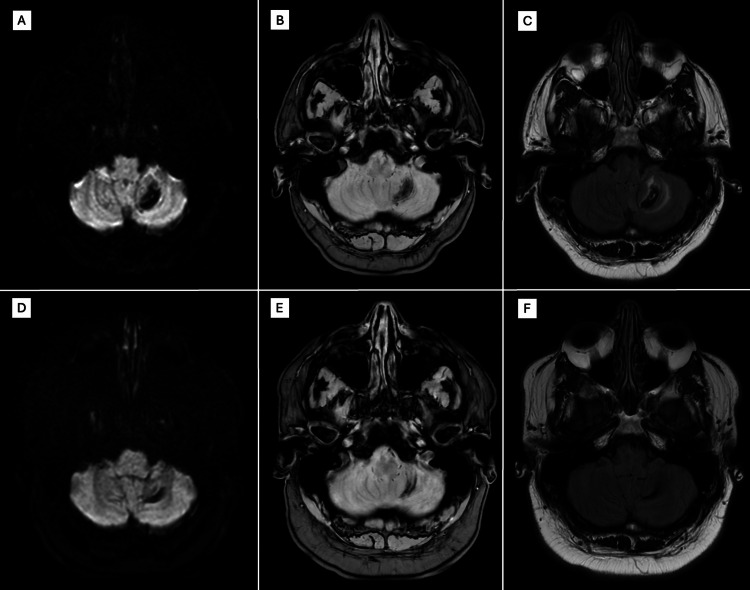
Cerebellar hemorrhagic lesion of neurosarcoidosis Initial diffusion-weighted imaging (DWI) (A), gradient echo sequences (GRE) (B), and fluid-attenuated inversion recovery (FLAIR) (C) brain MRI sequences showed restricted diffusion, blood products, and vasogenic edema around the neurosarcoidosis lesion in the left cerebellar hemisphere. Follow-up brain MRI at six-month follow-up with DWI (D), GRE (E), and FLAIR (F) sequences showed improvement of hemorrhage and resolution of vasogenic edema.
